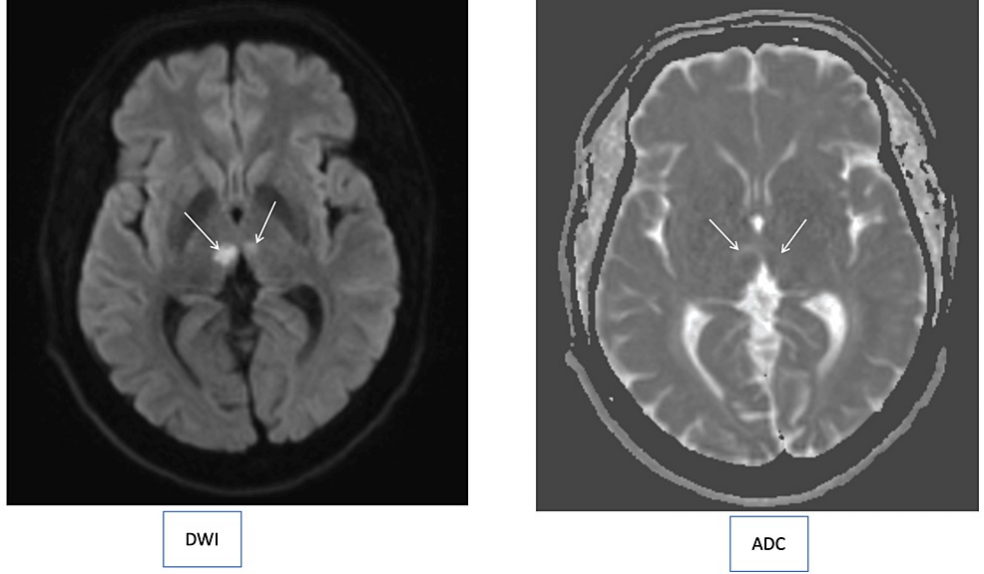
Bilateral medial thalamic area of diffusion restriction in DWI and ADC maps, larger on the right side compared to the left, consistent with acute ischemic lacunar infarcts along the AOP territory. DWI: diffusion-weighted imaging; ADC: apparent diffusion coefficient; AOP: artery of Percheron.
